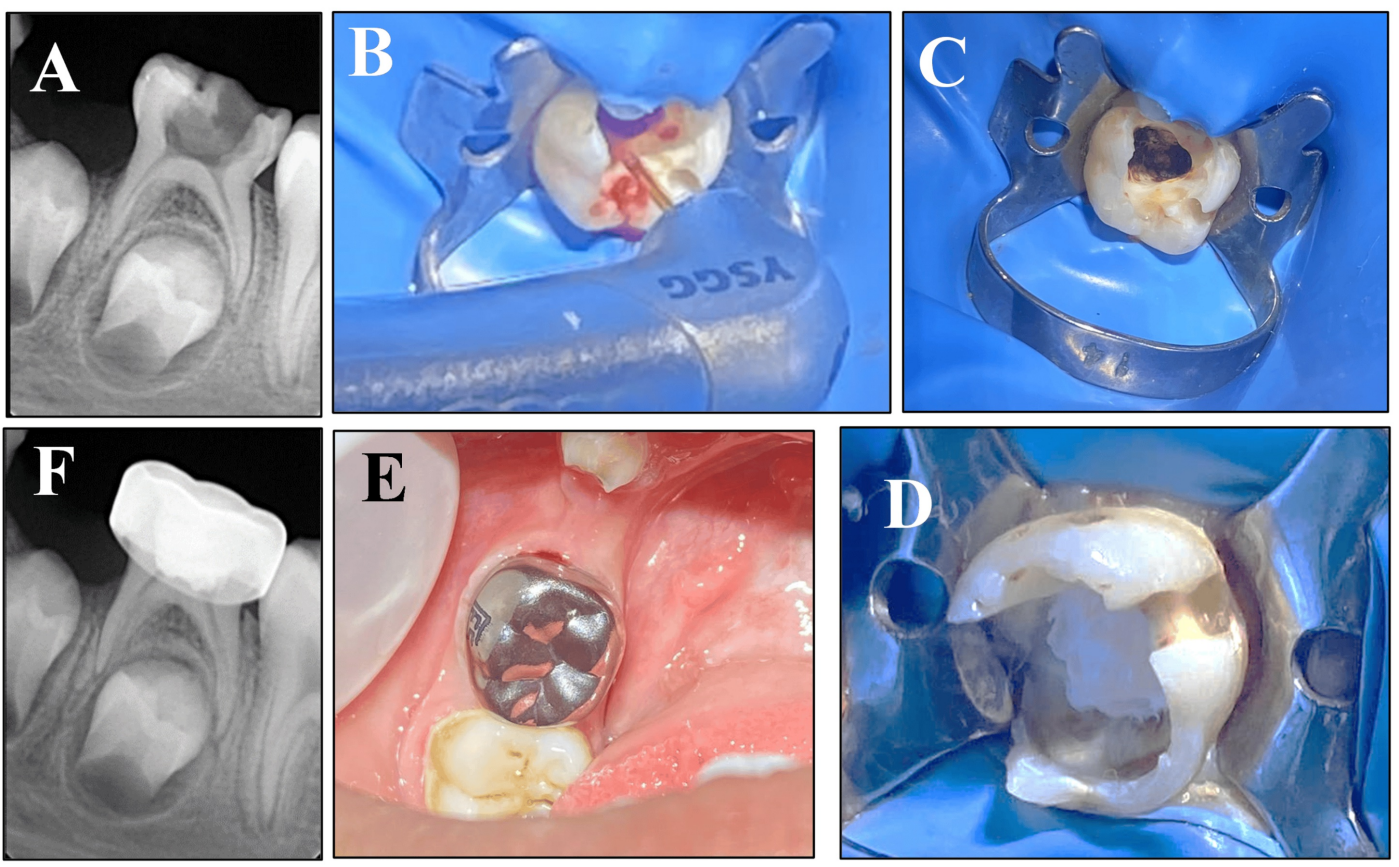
Sequence for the pulpotomy procedure using the Er,Cr:YSGG laser. (A) Preoperative periapical radiograph of a second primary molar showing an extensive caries lesion on the occlusal surface of the tooth. (B) Hemostasis of the pulpotomy sites using the Er,Cr:YSGG laser after the removal of the pulp chamber and pulp amputation. (C) Pulp stamps after laser application. (D) Application of a layer of the mineral trioxide aggregate (MTA) material to cover the pulpotomy site. (E) and (F) Clinical photograph and periapical radiograph showing the final restoration of the tooth.
